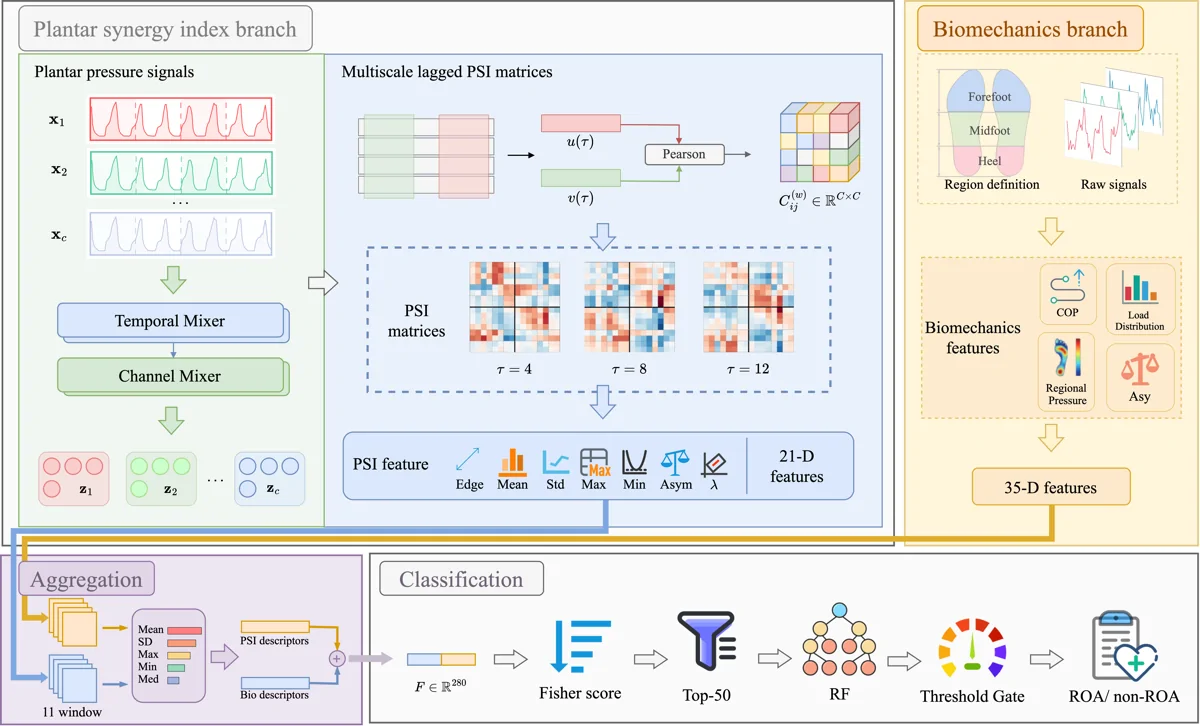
Two-branch plantar-pressure representation framework for subject-level ROA (radiographic knee osteoarthritis) identification. The biomechanics branch operates on raw pressure windows, whereas the PSI (Plantar Synergy Index) branch operates on the subject-wise standardized representation. Window-level features from both branches are aggregated to subject level for feature selection and final classification.
